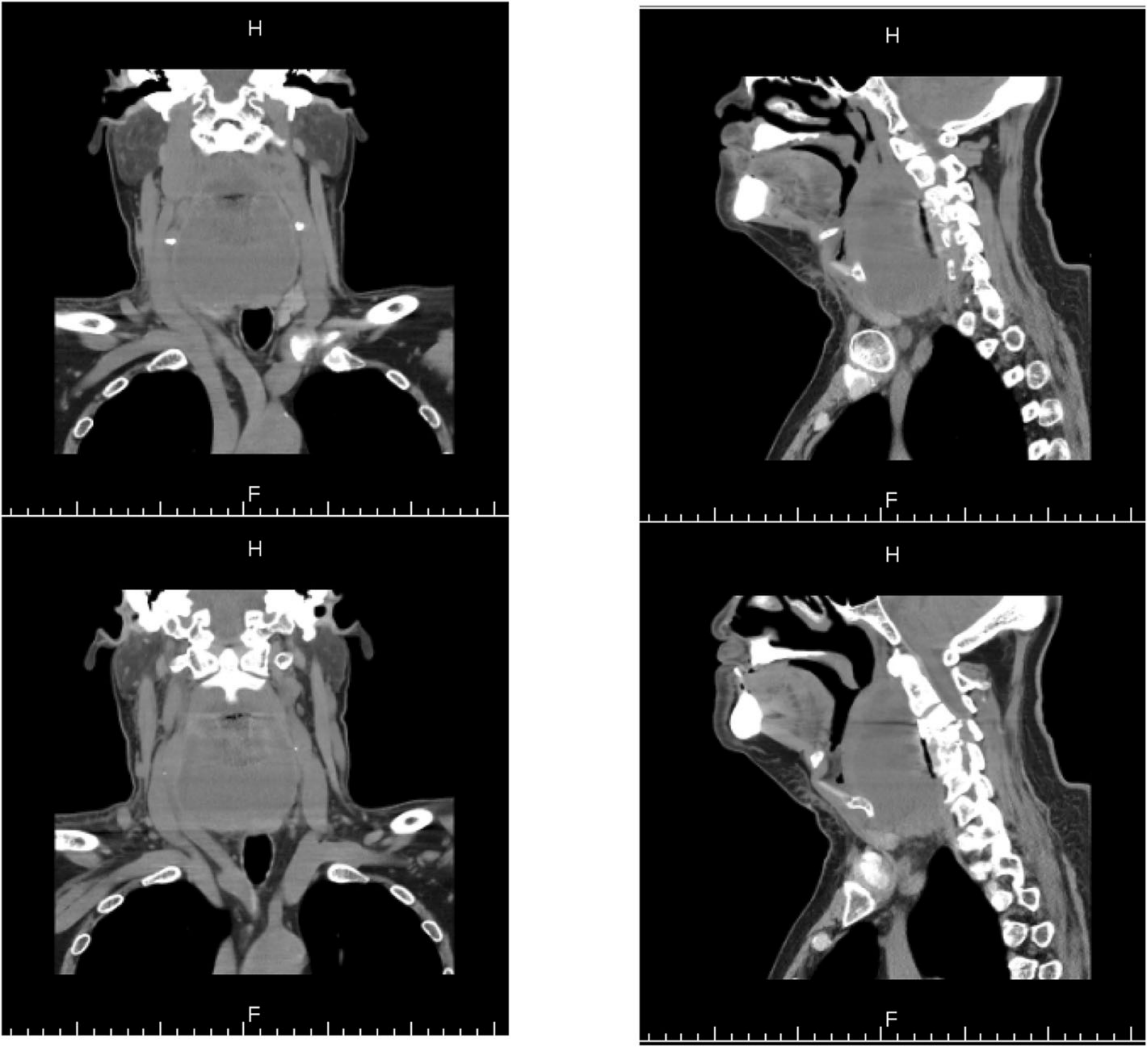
CT scan images on admission
